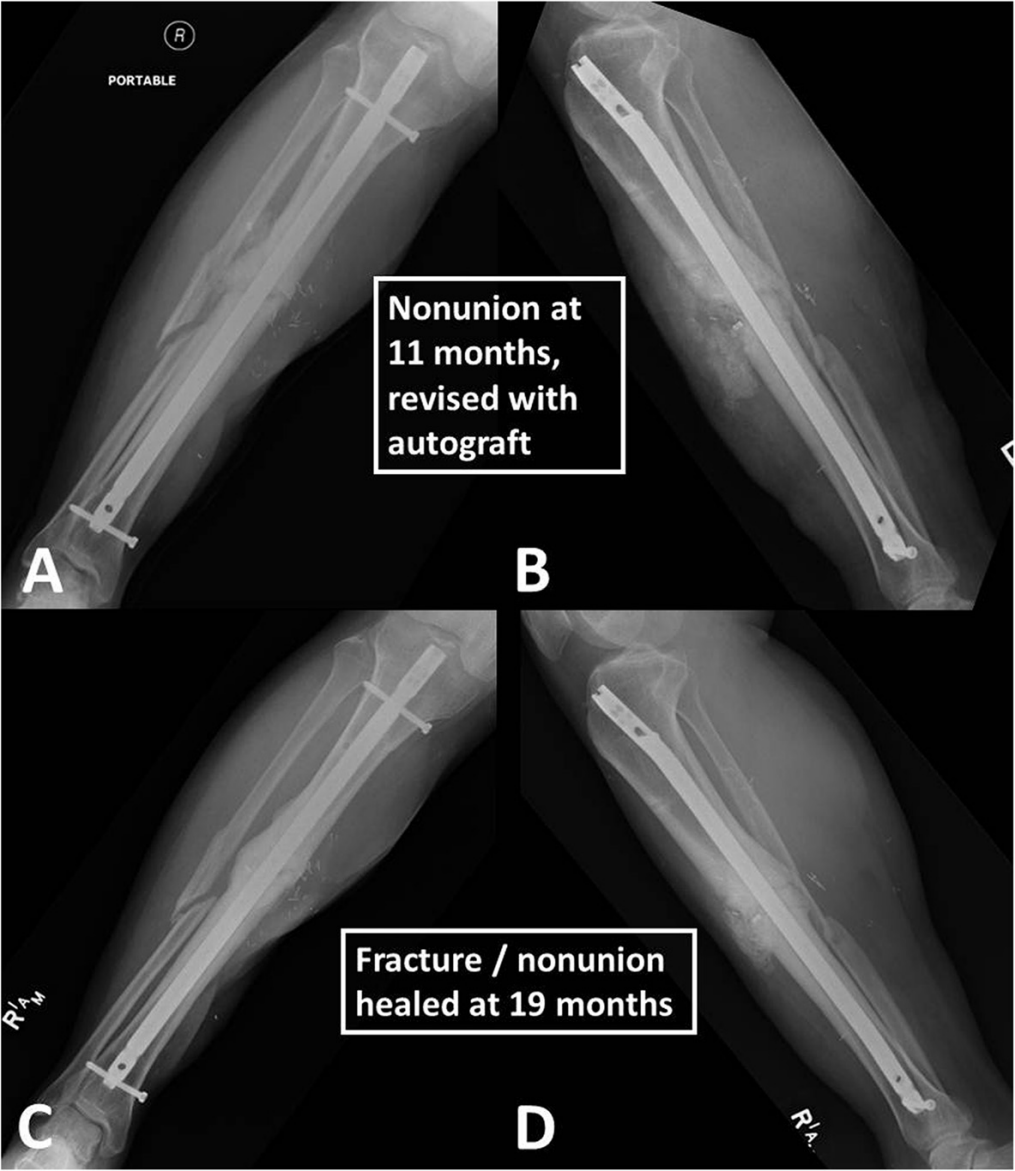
Identical case as in Figures2,3,4. The nonunion persisted at 11 months post injury, and a second revision was performed by decortication and application of a central bone graft (A, B), using a reamer-irrigator-aspirator technique for minimal invasive autograft harvest from the ipsilateral femur. The fracture/nonunion was clinically and radiographically healed at 19 months post injury (C, D), and the patient was asymptomatic with a full functional recovery.
